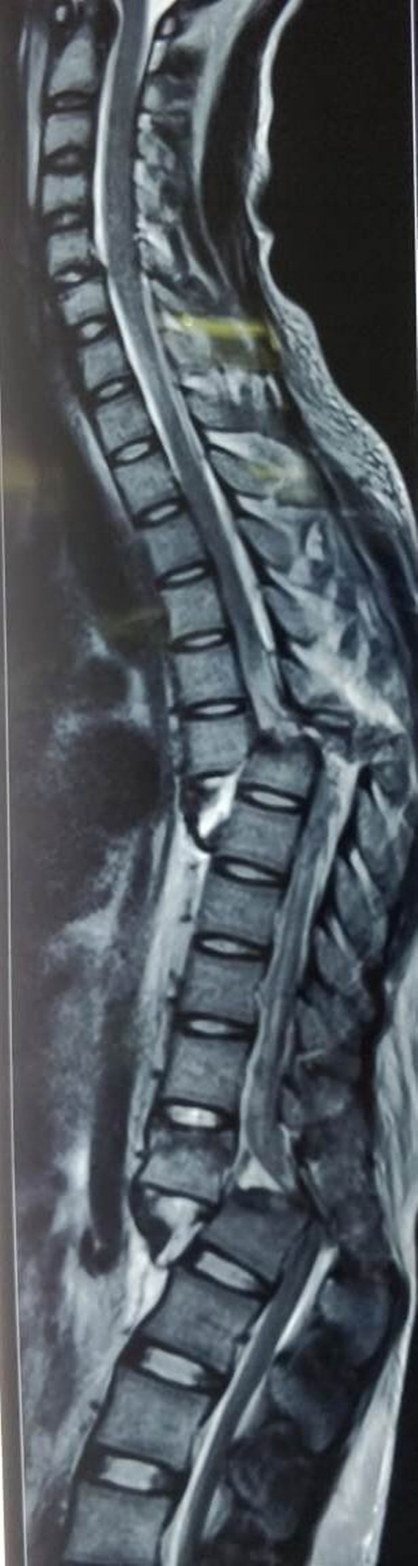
Preoperative sagittal T2-weighted MRI of spine showing the extent of damage to the spinal cord and posterior ligamentous complex
